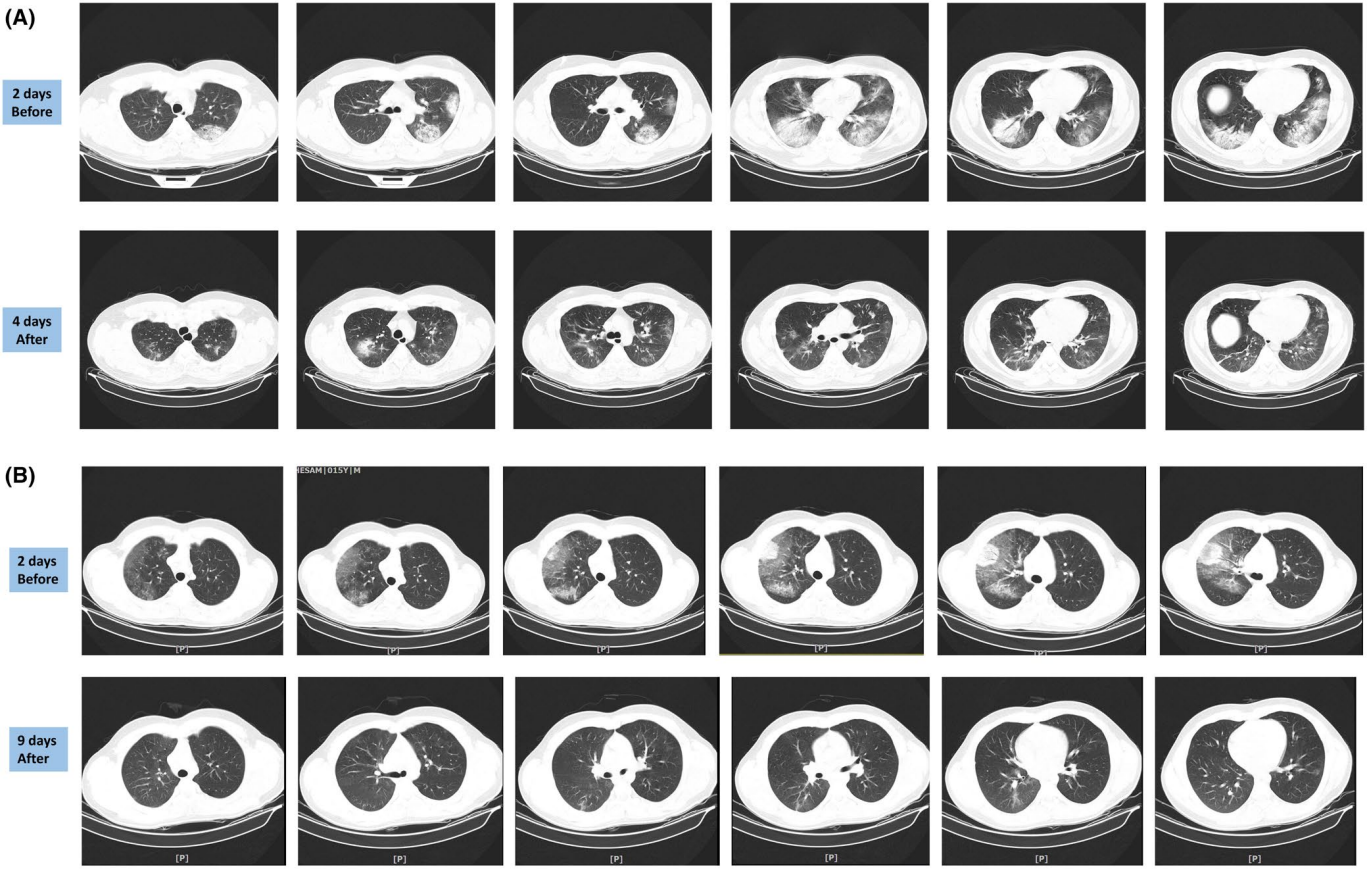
Computed tomography (CT) scan images of the lungs in patients with COVID‐19 infection before and after decidua stromal cells (DSC) therapy. (A) Lung CT scan of patient 9902, 2 days before and 4 days after DSCs treatment (1 day after the second DSC dose). For more information, refer to the text. (B) Lung CT scan of patient 9903, 2 days before and 9 days after DSC treatment (4 days after the second DSC dose). For more information, refer to the text. For a better overview of the changes, live CT scan images are available as Supplementary Files
